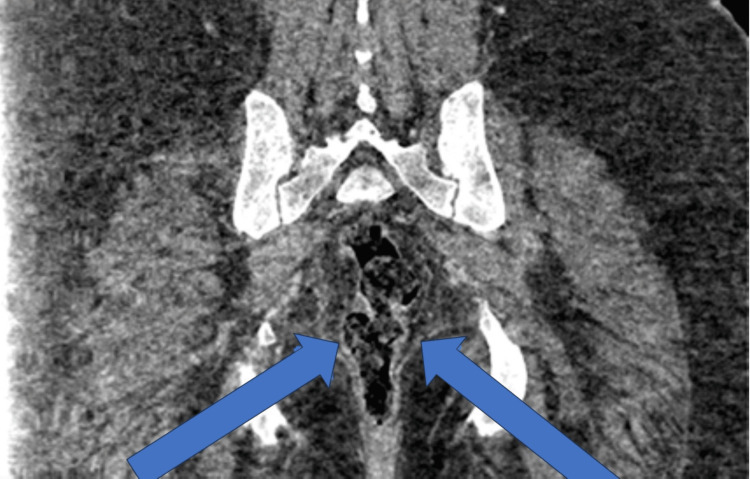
CT Neck showing the retropharyngeal phlegmon/abscess, Day 4 of admission Contrast‑enhanced CT revealing diffuse soft‑tissue swelling and retropharyngeal phlegmon extending into deep neck spaces without airway compromise
